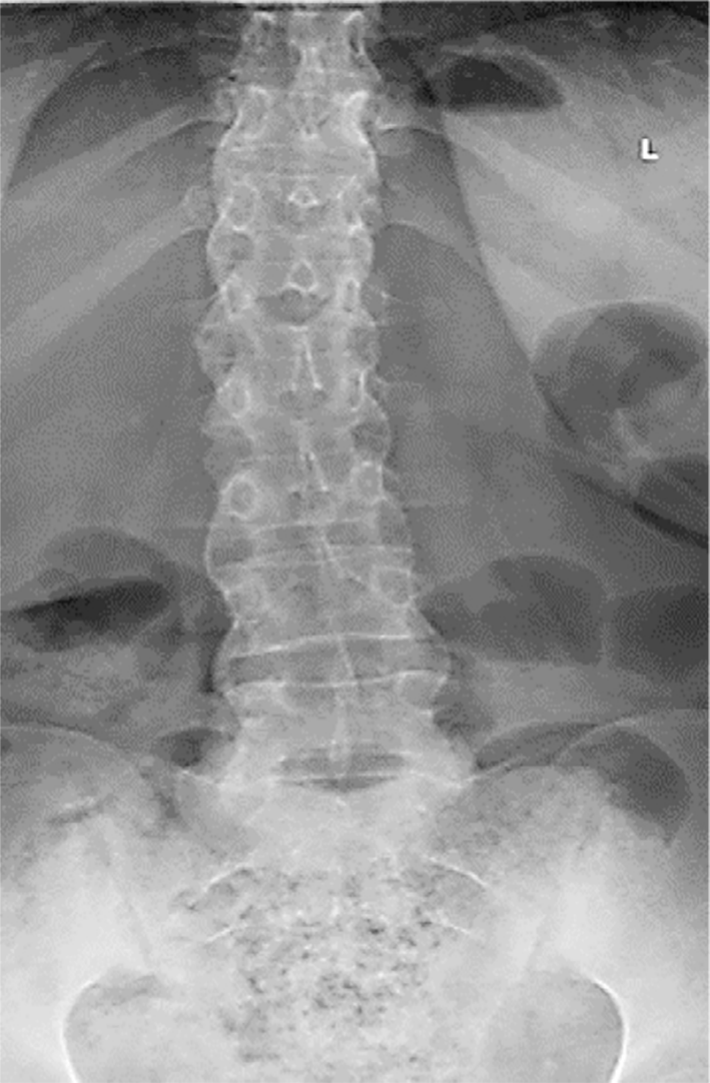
AP X-ray image demonstrating the characteristic bamboo appearance of the spine in a patient diagnosed with ankylosing spondylitis.
